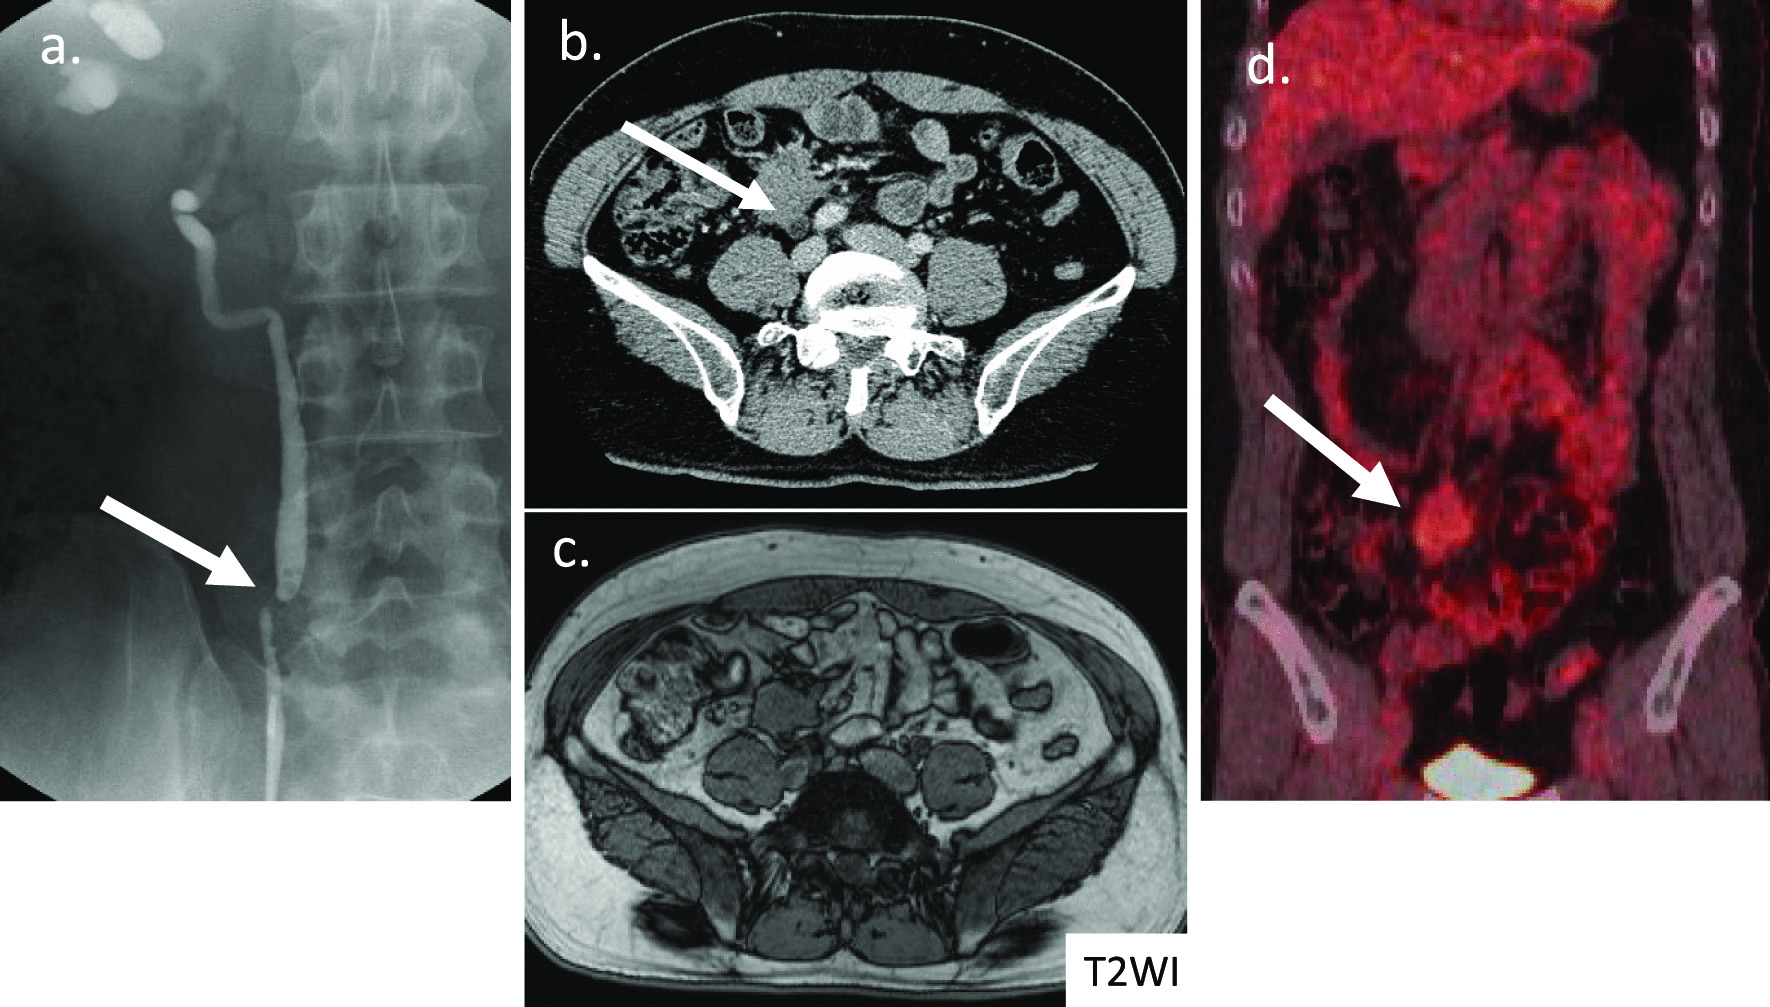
Diagnostic findings in Case 2. a Drip infusion pyelography displaying right uropathy stenosis (white arrow). b Abdominal contrast-enhanced computed tomography (CT) displaying a homogeneously enhanced 35-mm mass with an irregular, spiculated margin encasing the right dilated ureter at the level of the right common iliac artery (white arrow). c T2-weighted image displaying a low signal mainly at the center of the tumor. d
